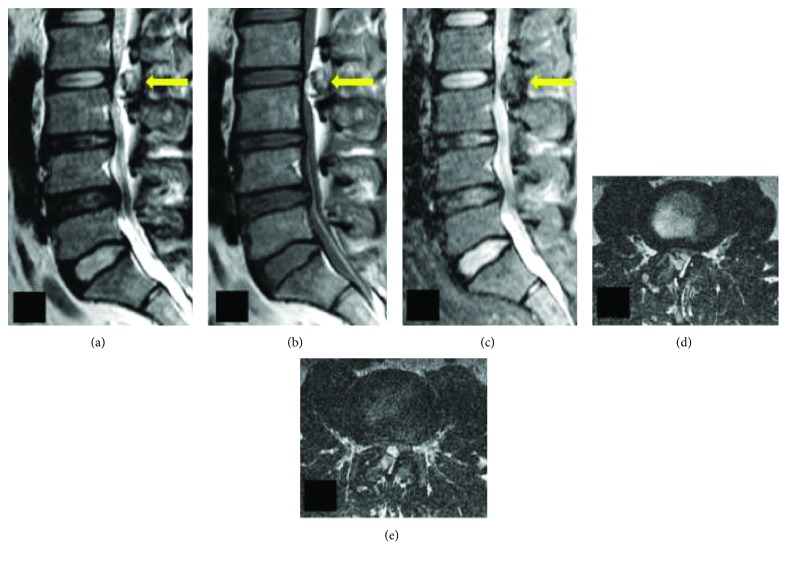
(a and b) T2- and T1-weighted sagittal MRI images showing a large epidural intraspinal posterior epidural lesion with hyperintense signals in the periphery and hypointense signals at the center at the L2-L3 level with severe thinning of the thecal sac. The hyperintense region becomes hypointense in the fat-suppressed sequence (c) confirming the presence of fat in the periphery and bone tissue in the center of the lesion. (d and e) Axial images demonstrating adjacent epidural fatty hypertrophy contributing to stenosis in addition to the lesion reducing the caliber of dura to near nonexistence.
